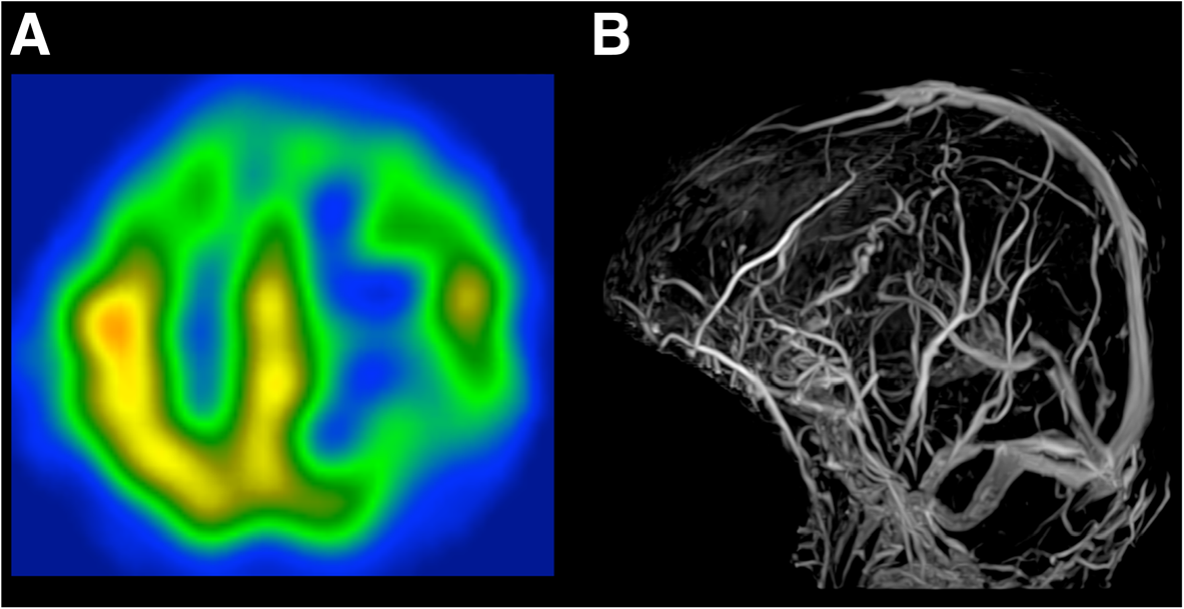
Extended areas of hypoperfusion in the entire left hemisphere and right frontal lobe were observed by p-iodoamphetamine-single photon emission computed tomography (IMP-SPECT) (a). Magnetic resonance venography revealed no significant occlusive regions in the cerebral venous system (b)
